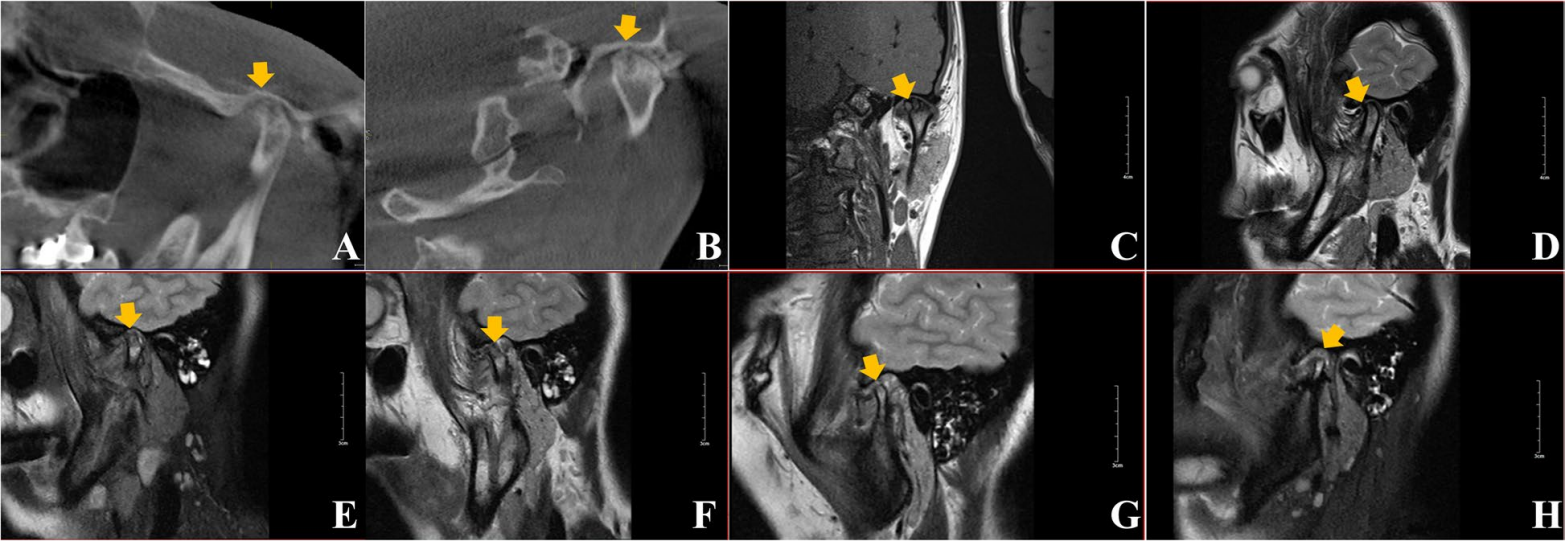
Preoperative (A-D) and postoperative (E-H) radiographic results. Fig. A was visualized by oblique sagittal position of cone beam computed tomography (CBCT), B was oblique coronal position of CBCT image; Fig. C was visualized by oblique coronal position of magnetic resonance imaging (MRI), D was oblique sagittal position of MR image. Figs. E, F, G, and H were MRI oblique sagittal slices, with the performance of animate muscle tissue or/and accompanied by adipose tissue indicated by the graft of temporalis myofascial flap in joint space
